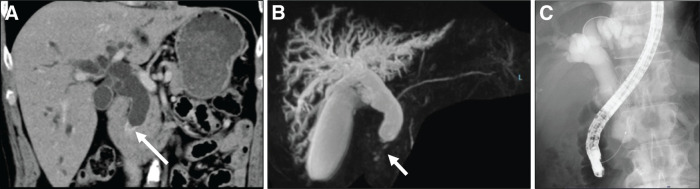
Preoperative imaging at the time of the initial surgery. (A) Contrast-enhanced abdominal CT demonstrating wall thickening of the distal bile duct. The arrow indicates the thickened distal bile duct. (B) MRCP showing dilatation of the common bile duct and intrahepatic bile ducts. The arrow indicates the dilated common bile duct. (C) ERCP revealing stenosis of the distal bile duct with upstream biliary dilatation. ERCP, endoscopic retrograde cholangiopancreatography; MRCP, magnetic resonance cholangiopancreatography
